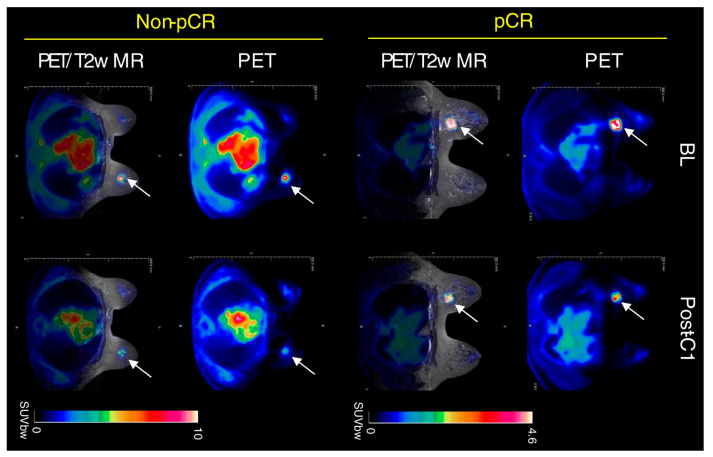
Clinical PET/MR images of response to therapy in triple-negative BC after NAC. A tumor is indicated by a white arrow. Adapted from Roy S et al. [44] published under a Creative Commons Attribution 4.0 International License http://creativecommons.org/licenses/by/4.0/, accessed on 27 June 2023.
